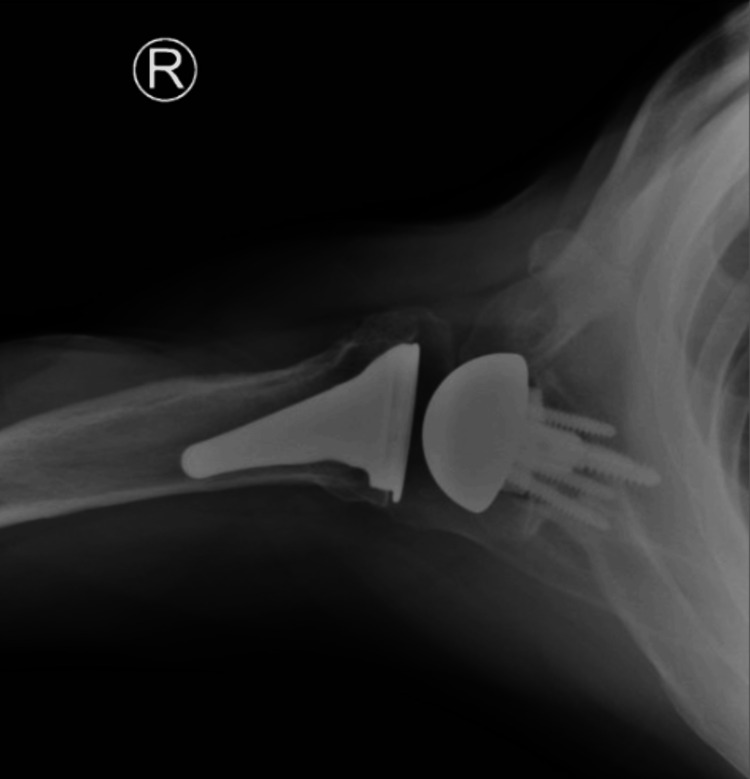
Postoperative X-rays of the right shoulder at one-year follow-up The image depicts an axillary view of the right shoulder
